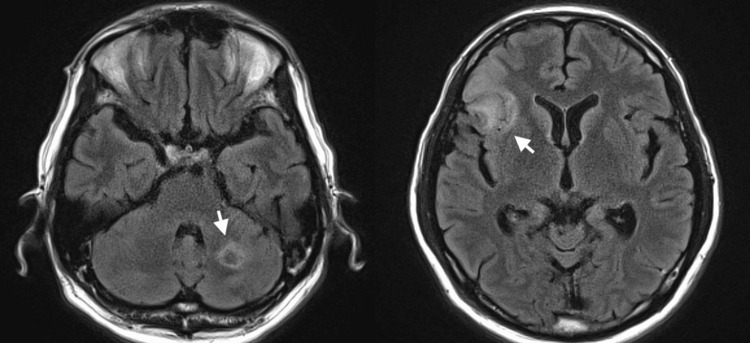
Multiple cerebral infarcts on brain MRI Complications of *Abiotrophia defectiva* infective endocarditis.
